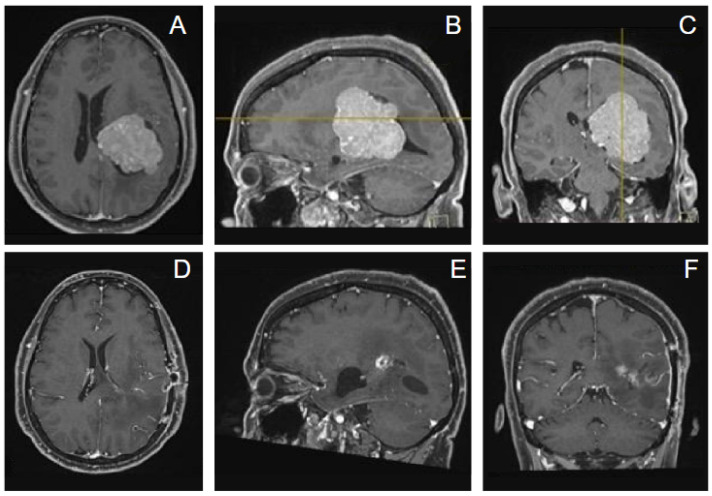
The axial (A), sagittal (B), and coronal (C) contrast-enhanced preoperative MRI showed a large intraventricular mass in the left trigone with peritrigonal extension. (D–F). Contrast-enhanced postoperative MRI revealed a complete tumor resection, with minimal brain damage.
